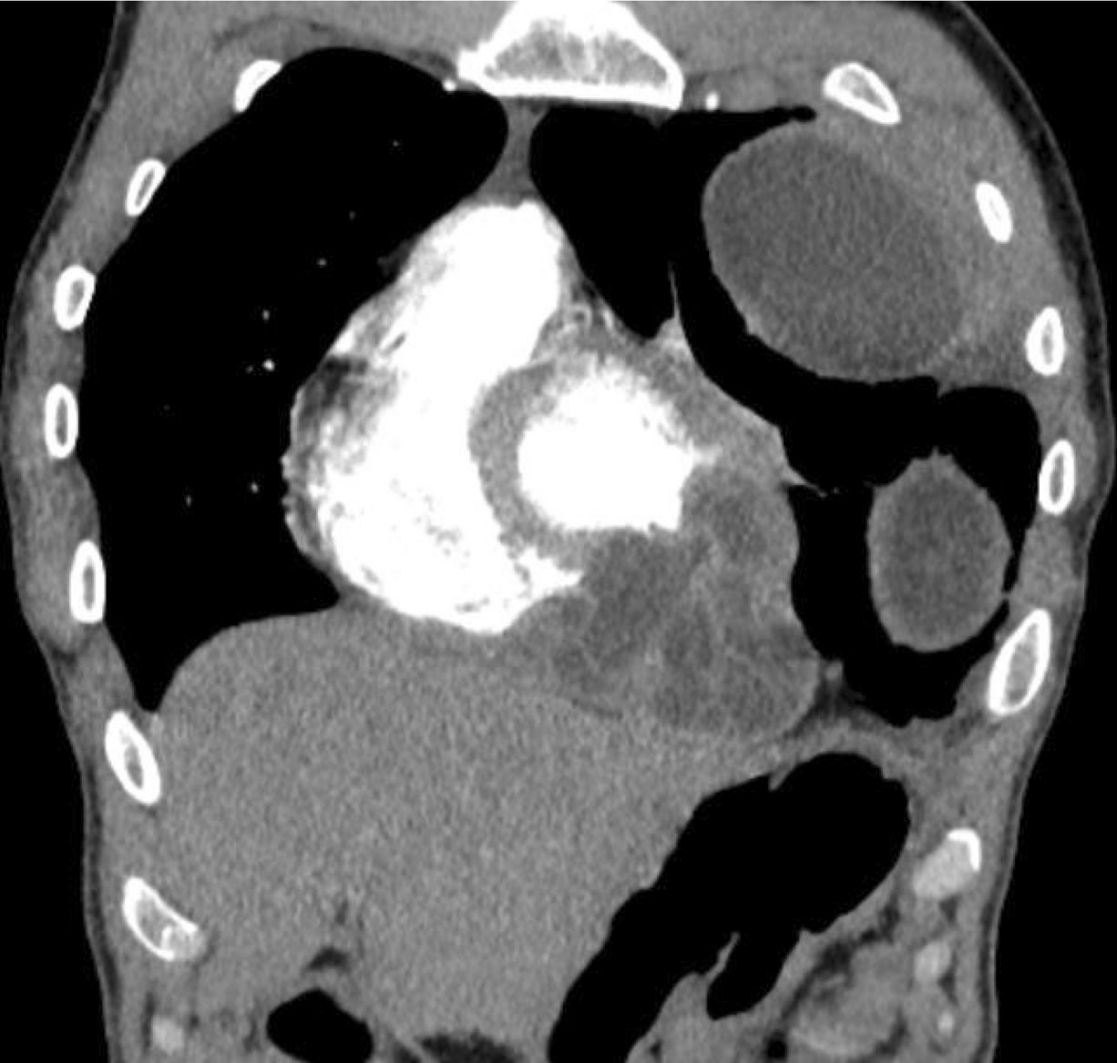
Contrast enhanced chest CT, coronal section: A multi-Septate, fluid attenuating cystic lesion in the cardiac apex
